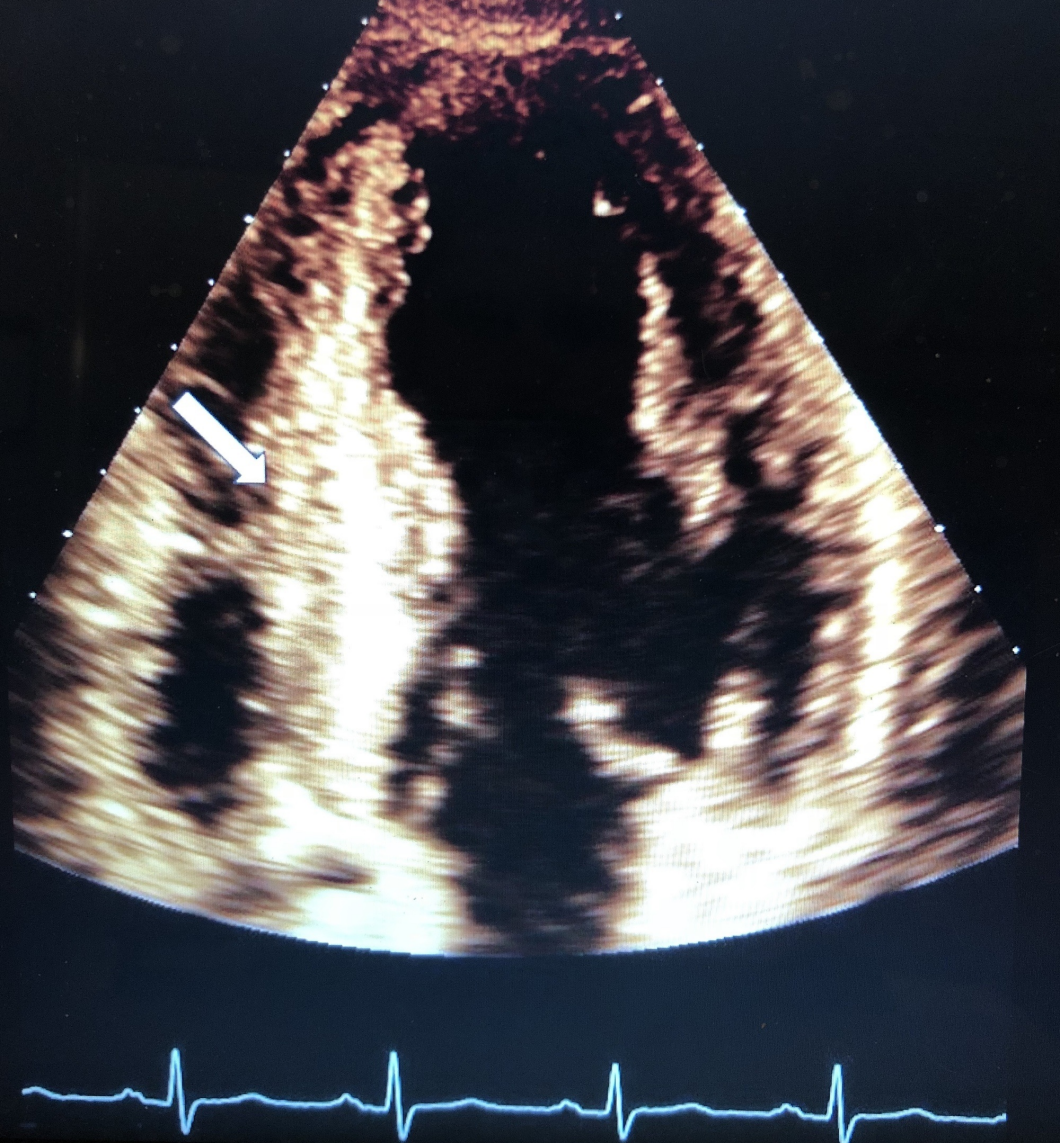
Apical four-chamber view with arrow pointing to hypertrophied septum
